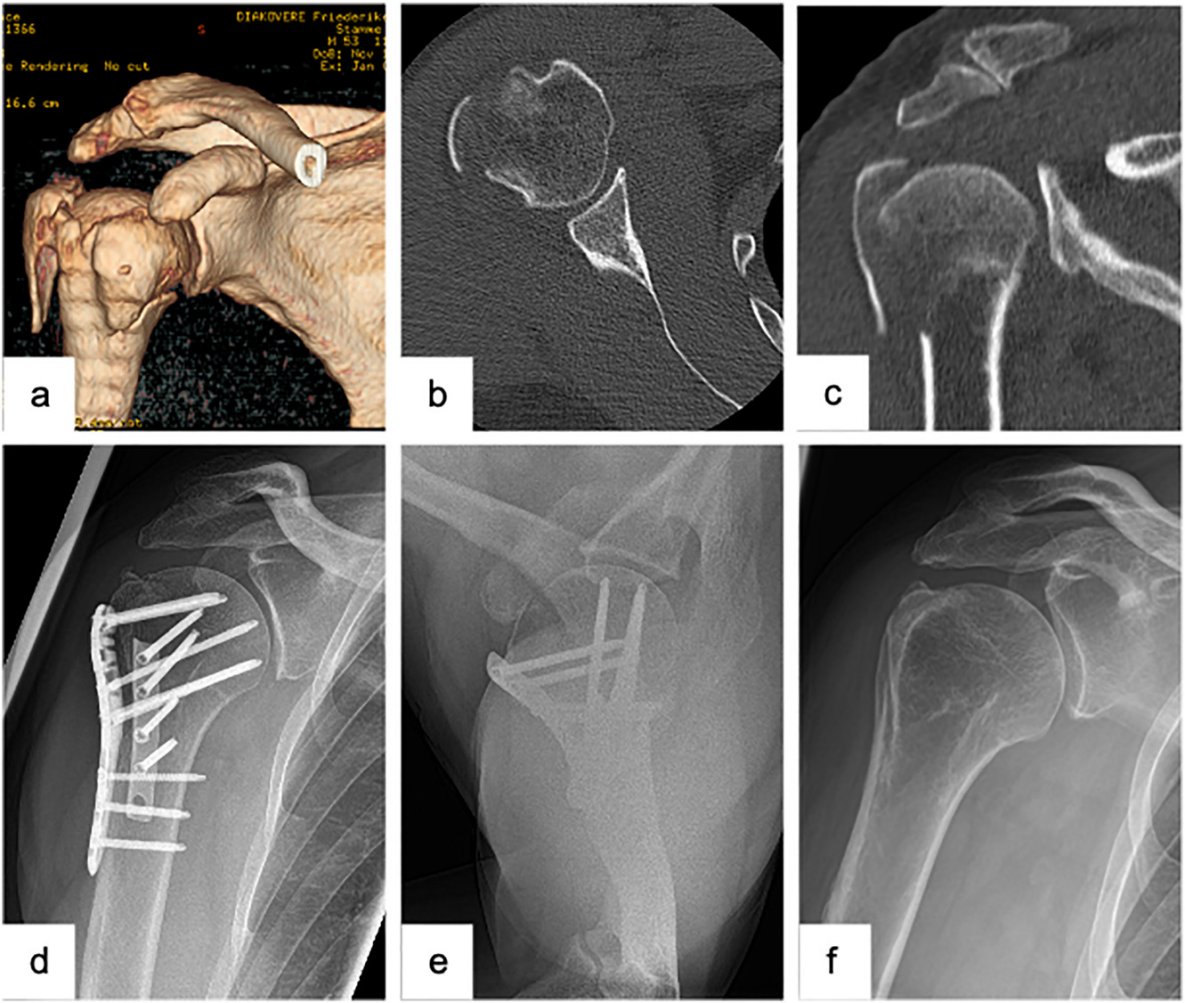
Male patient (53 y), right shoulder, valgus impacted 4-part proximal humerus fracture (preoperative (3D-) CT-scan: a – 3D-CT-reconstruction, b – axial, c – coronal), open reduction and internal fixation with a laterally positioned locking plate osteosynthesis and a second, supportive, ventrally positioned plate to stabilize the lesser tuberosity (postoperative X-ray: d – a.p., e − Y-plane), complete removal of the double plate osteosynthesis and arthroscopically assisted arthrolysis (postoperative X-ray: f – a.p.).
